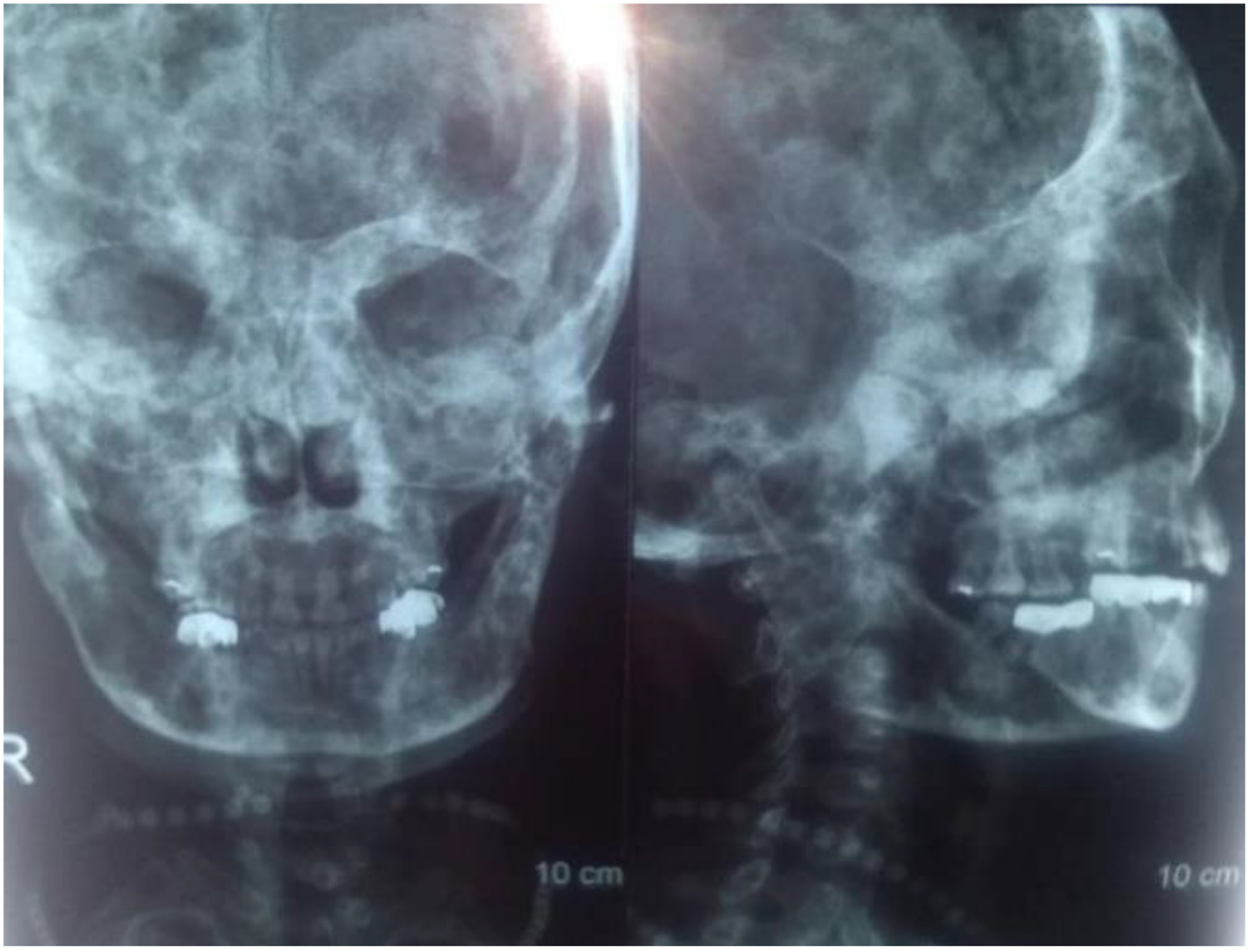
X-rays of osteonecrosis of the mandible.
